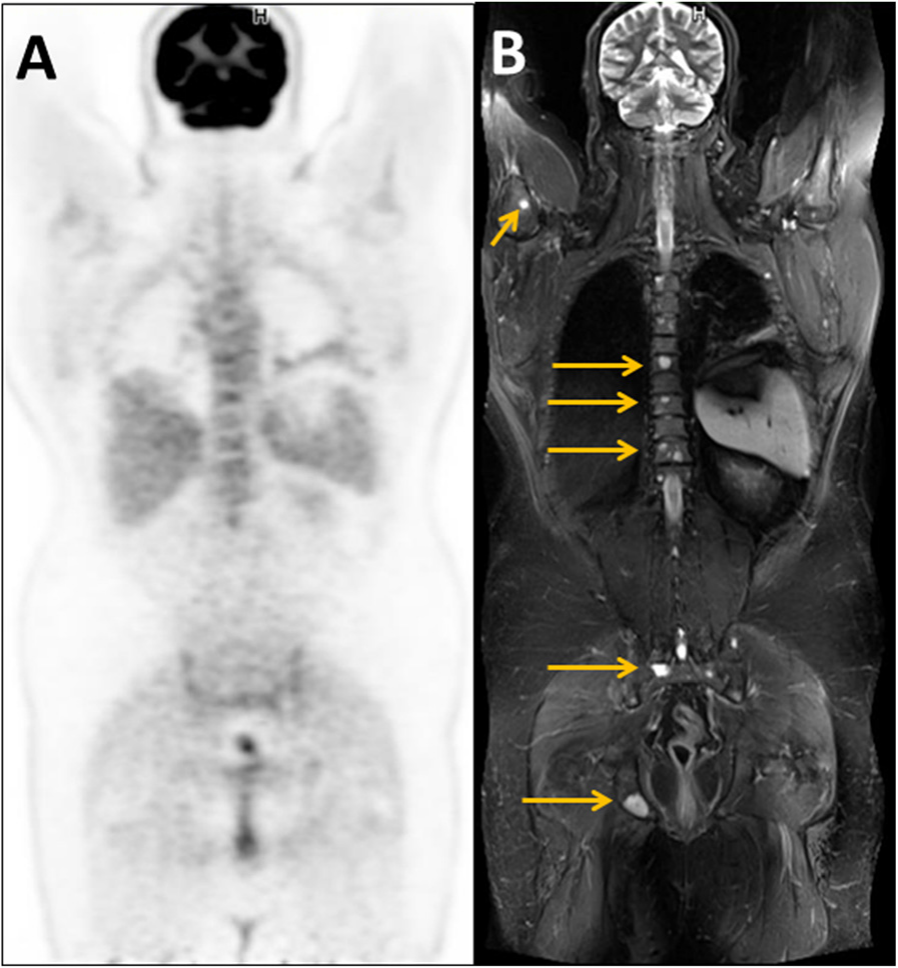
PET/MRI study comprising whole-body FDG-PET (a) and Short Tau Inversion Recovery (STIR) MRI (b) from a patient with recurrent myxoid liposarcoma. The skeletal metastases are more readily appreciated on the MRI than on FDG-PET
